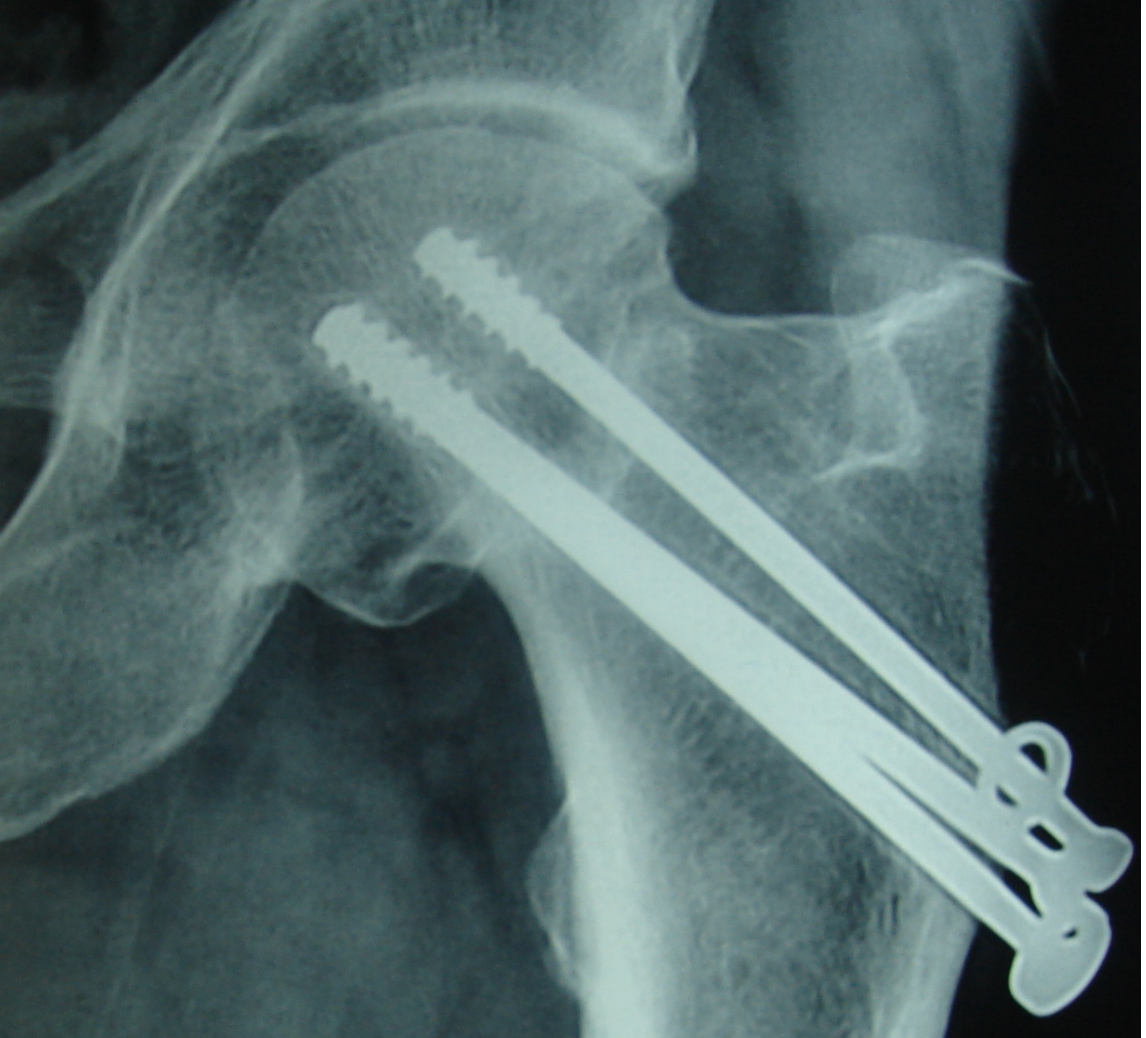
Case no 2, radiograph showing union at 29 months.
